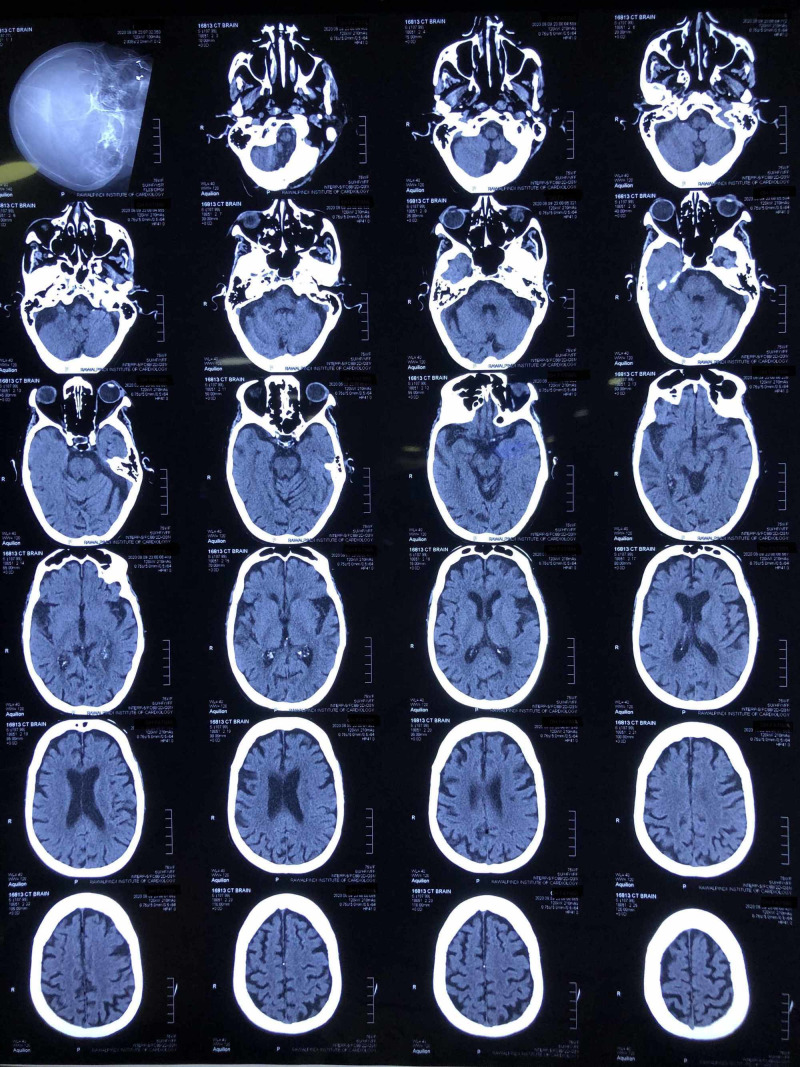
CT scan brain of the patient
